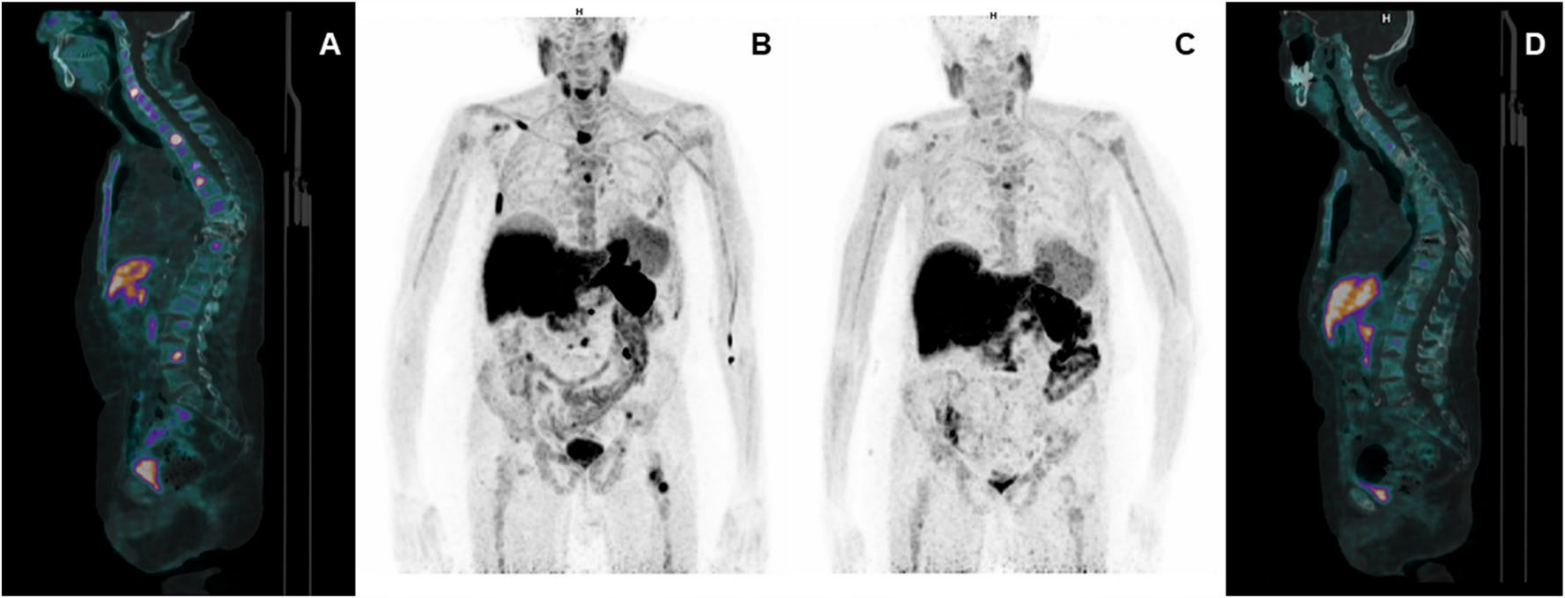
Baseline [18F]F-choline PET/CT scan (A sagittal fused image and B maximum intensity projection) showed several skeletal PCa pathological uptakes. Post-treatment [18F]F-choline PET/CT scan (C maximum intensity projection; D sagittal fused image), performed after [223Ra]RaCl_2_ therapy showed a significant reduction of metastatic bone uptakes (i.e. SUVmax decreasing from 19.3 to 5.4 on the left femoral neck lesion)
